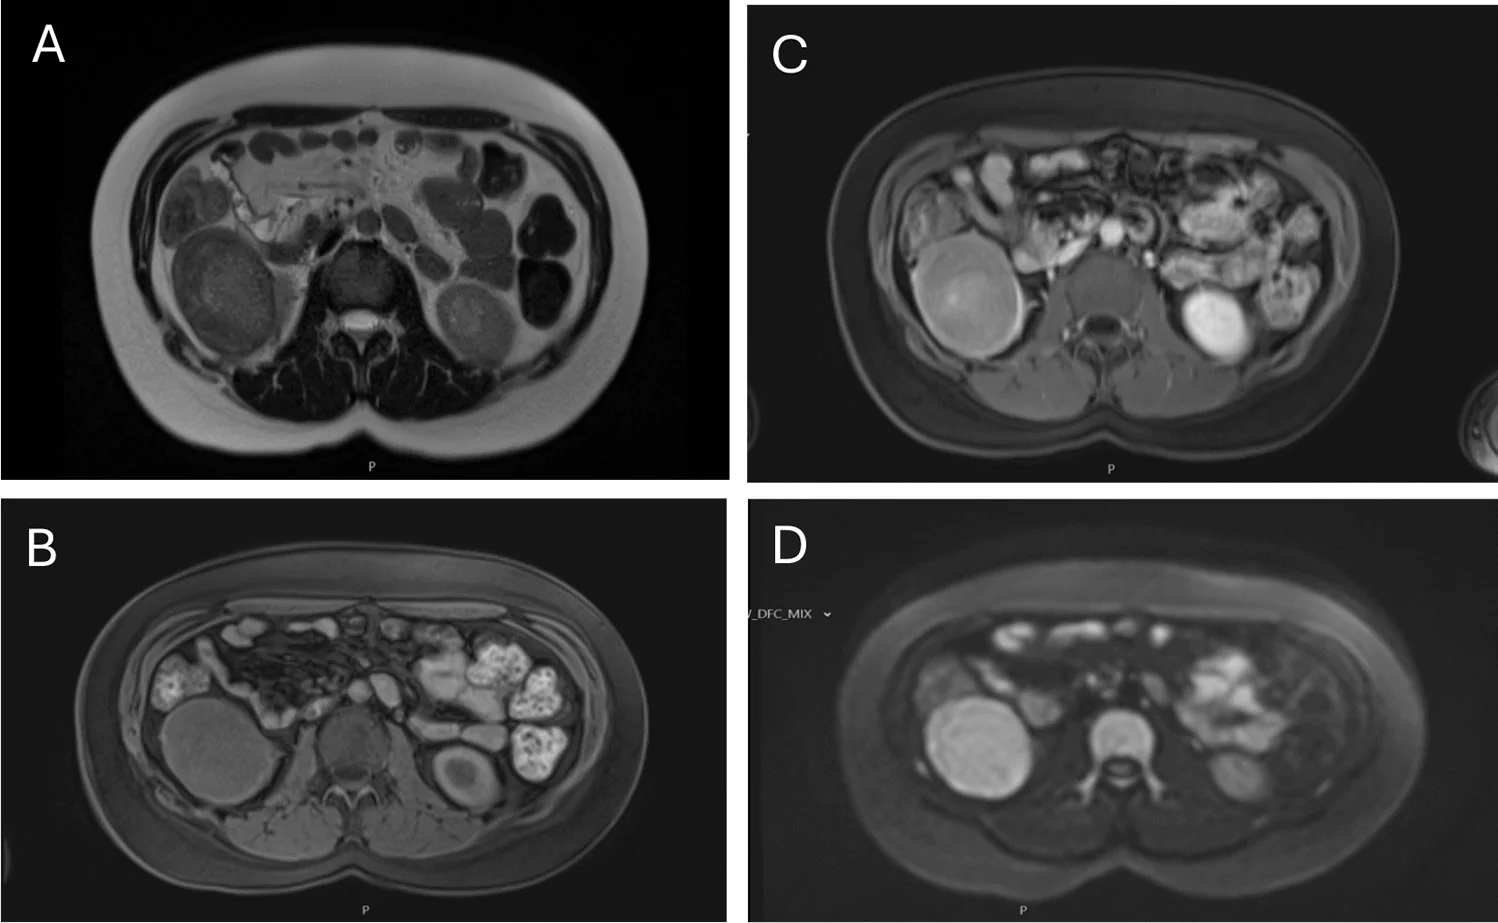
29-year-old Middle Eastern female without sickle cell trait presenting with abdominal pain. A Axial T2-weighted (T2w), B fat-saturated non-contrast-enhanced T1-weighted (T1w), C contrast-enhanced T1-weighted, and D b800 diffusion-weighted MRI images show a well-defined exophytic right renal mass. It is heterogeneous on T2w, isointense on T1w, and hypoenhancing relative to the cortex. The mass effaces the renal sinus fat and lower pole calyces, with restricted diffusion (ADC map not shown)
